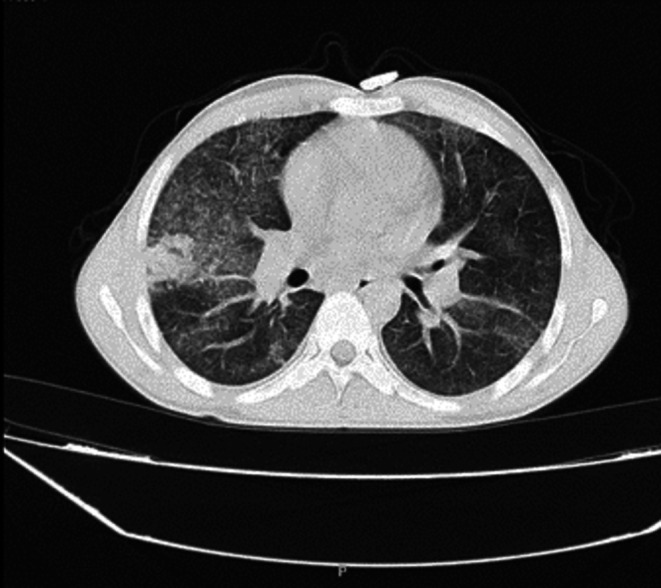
HRCT showed pulmonary infection.
